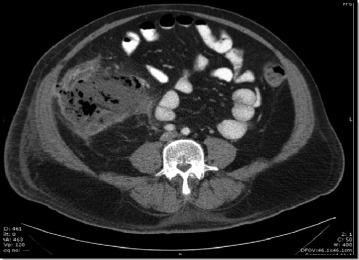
The CT scan of abdomen showing an inflammatory tumor in the lower right quadrant. The patient was managed with antibiotics only; i. e non-operative treatment. (Grade 3A)
